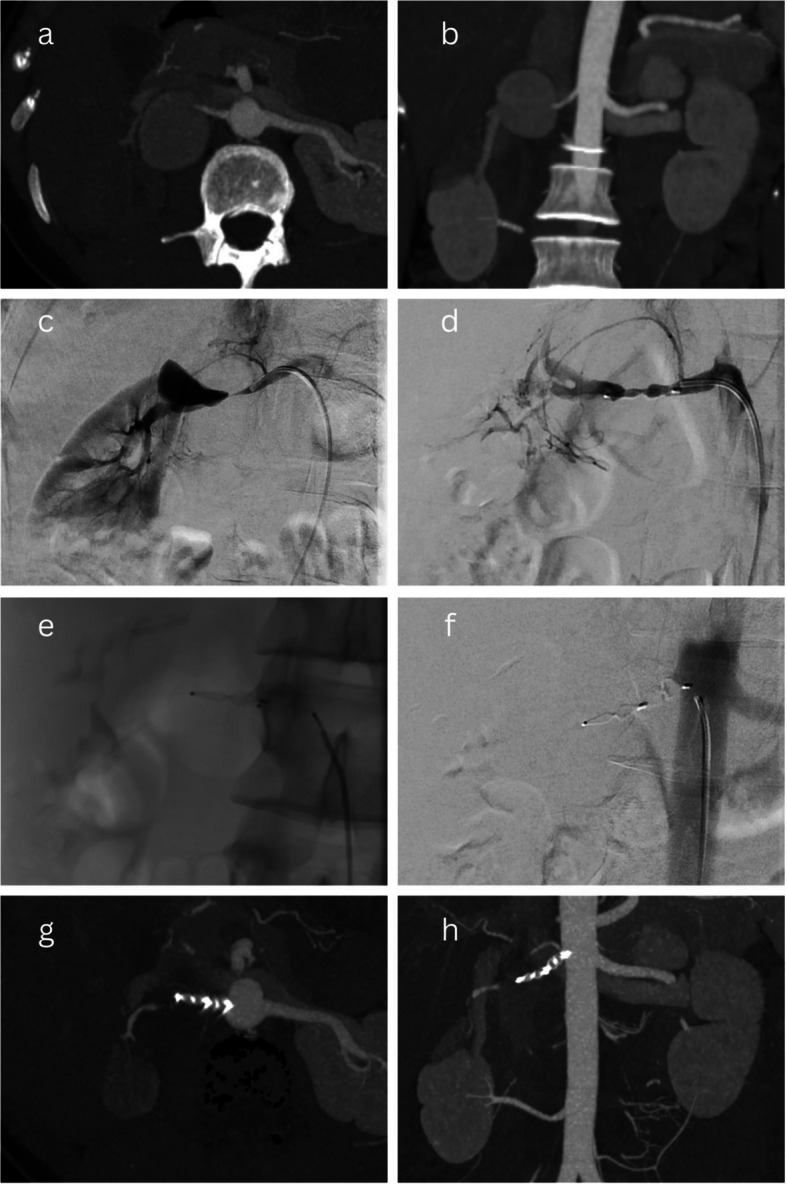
Plug placement in proximal main artery of a TRAA. A saccular Type 1 Rundback aneurysm was treated with plug placement in the proximal main artery supplying the TRAA as it was already associated with distal renal ischaemia (a-b) and the presence of renal artery stenosis also limited access to the sac (c). A wire was manipulated into the sac and a vascular plug was deployed at the proximal TRAA neck (d). A further vascular plug was deployed in the proximal main renal artery up to its origin (e). Post-treatment angiography confirmed successful exclusion of the aneurysm (f). A follow up CT angiogram at 8 weeks confirmed persistent occlusion of the aneurysm (g-h)
